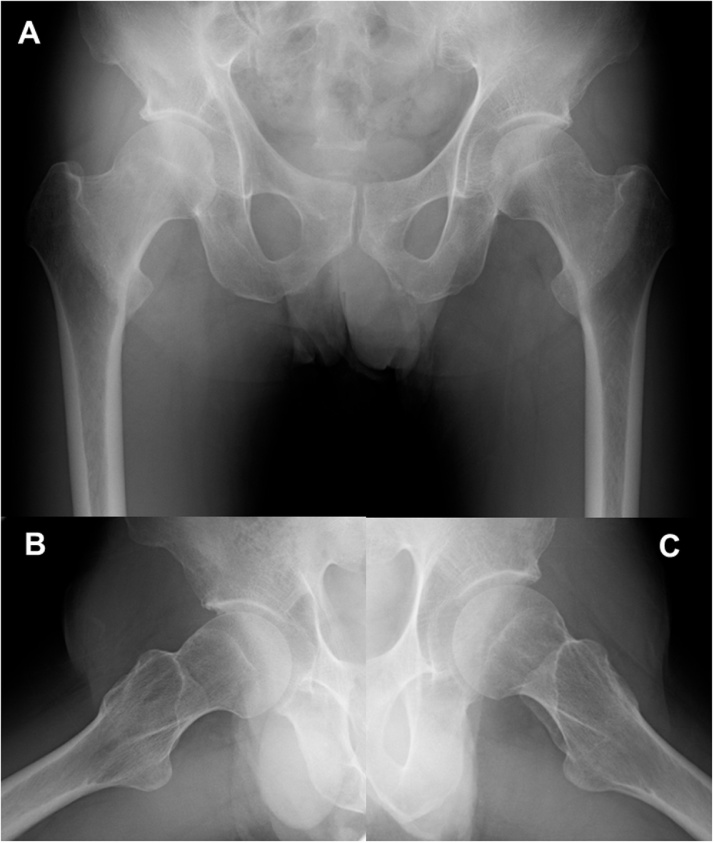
(A) Initial anteroposterior radiograph shows no significant findings except for mild pistol grip deformity on right hip and (B,C) small head-neck offset compared with left hip.
